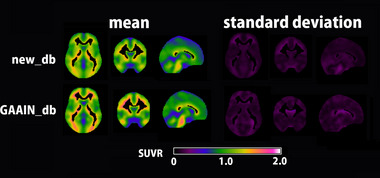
Standardized uptake value ratio (SUVR) images for the mean and standard deviation of the present new database and the Global Alzheimer's Association Interactive Network (GAAIN) database. In the target cortical volume of interest (VOI), the SUVR values (0.986 ± 0.052) for the present new database (new_db, 13 men and 7 women; 45.4 ± 3.9 years old) are significantly (*p* < .005) lower than those (1.048 ± 0.064) for the GAAIN database (GAAIN_db, 7 women and 6 men; 27.0 ± 4.3 years old).
